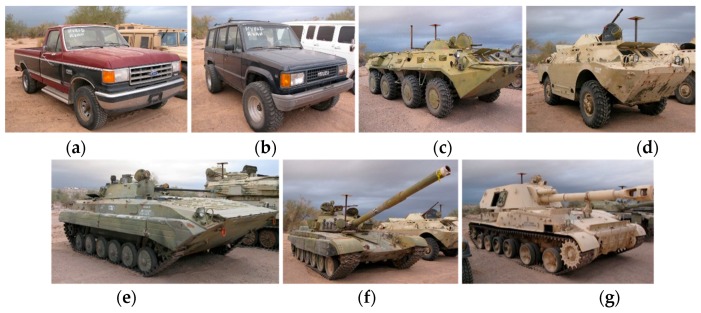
Seven targets in SENSIAC: (a) Truck; (b) SUV; (c) BTR70; (d) BRDM2; (e) BMP2; (f) T72; and (g) ZSU23-4.
